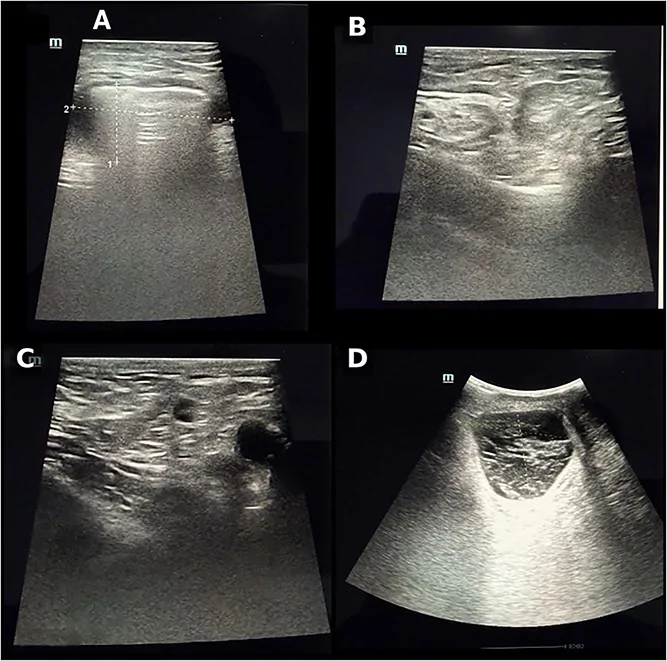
High-frequency linear-transducer soft-tissue ultrasound of the right inguinal region; (A) measurement of the herniary defect in the anterior abdominal wall, lateral to the epigastric vessels (55 × 63 mm); (B) heterogeneous fatty content and bowel loops within the sac, extending to the distal third of the inguinal canal; (C) herniary contents with hypoechoic foci compatible with intestinal gas; (D) convex-transducer view of a dilated bowel loop with mixed content inside the sac; the defect was neither reducible with directed transducer pressure nor modifiable with the Valsalva manoeuvre, without surrounding inflammatory changes.
